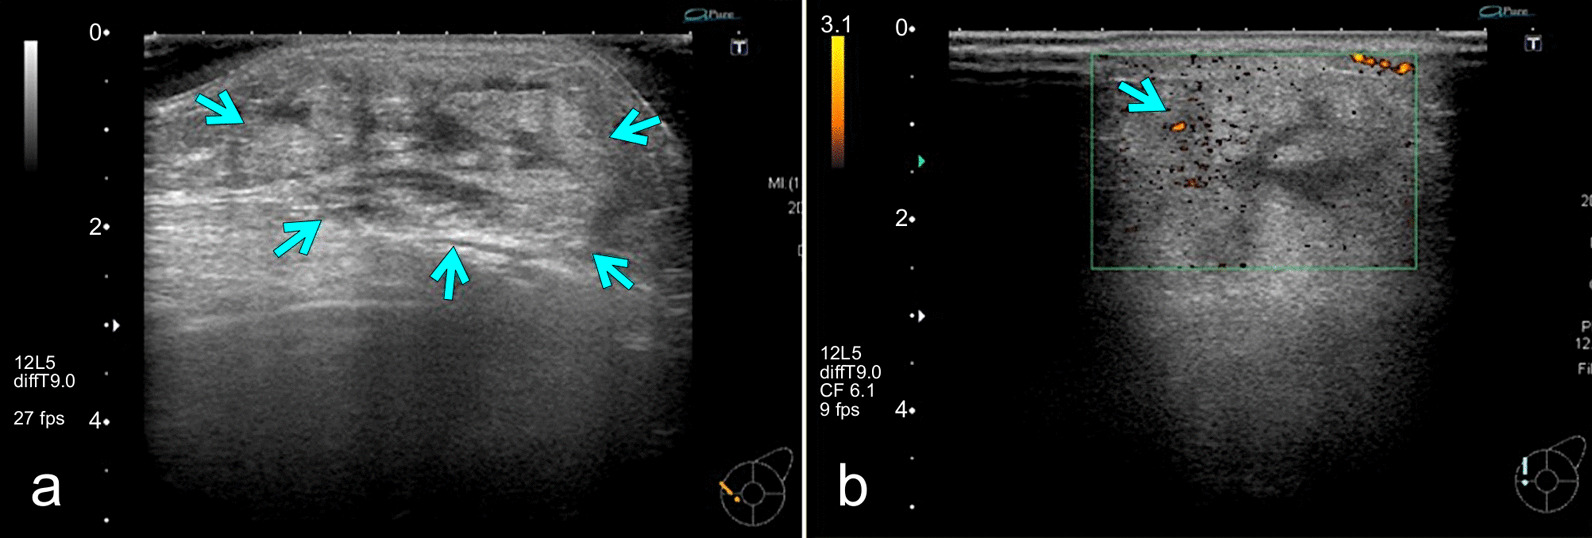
Ultrasonography (US) of the left breast. One week after withdrawal of methotrexate, a US showed a 4.5-cm heterogeneous hyperechoic mass in the internal region of the left breast (arrow). b The mass had a low echo area spread like a cord, and blood flow was partially observed along the low echo area (arrow)
